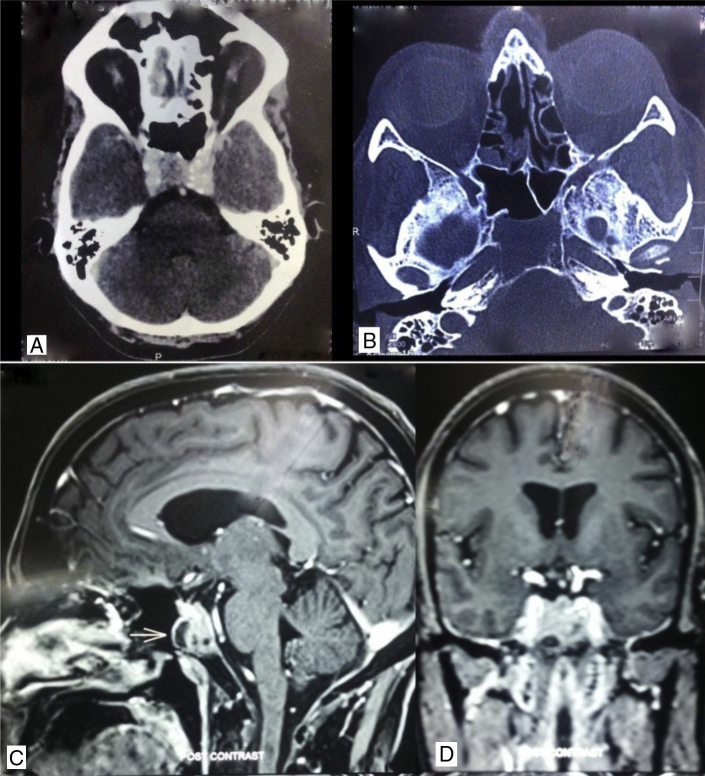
(A) Contrast enhanced computerized tomography axial view showing brilliantly enhancing mass involving the clivus. (B) Computerized tomography – axial view, bone window, showing osteolytic lesion of clivus. (C) Magnetic resonance imaging – sagittal view showing clival lesion with hypoechoic area. (D) Magnetic resonance imaging – coronal view.
